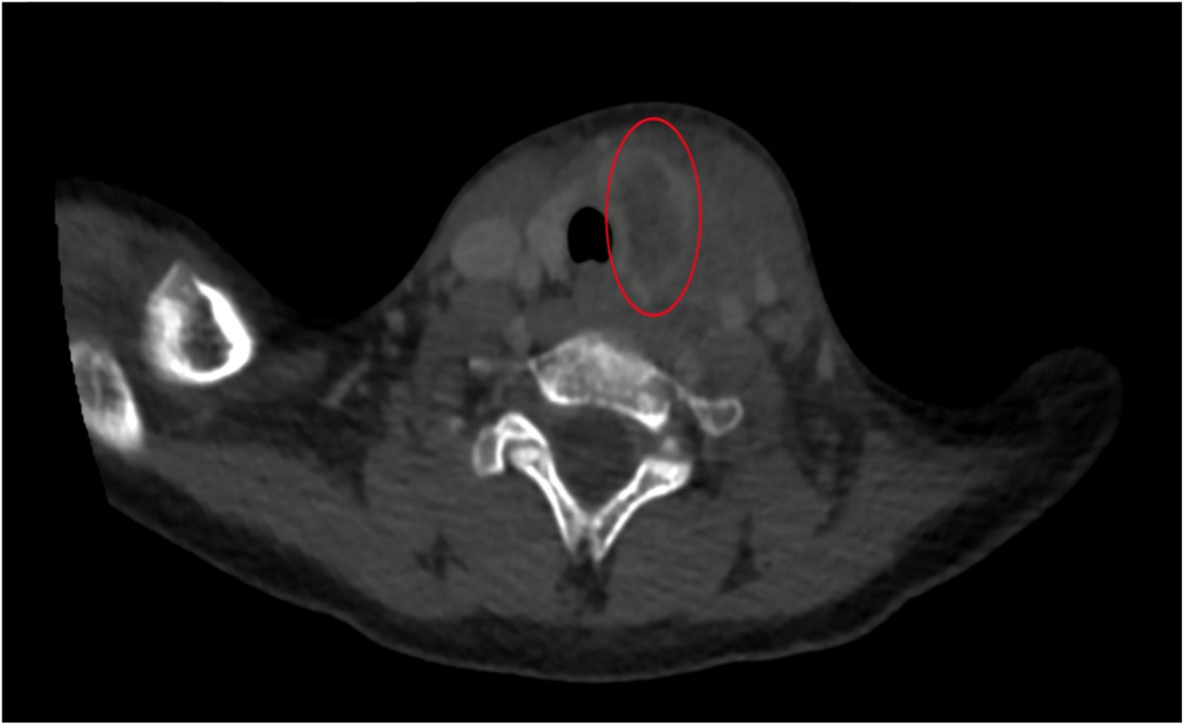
CT scan of neck depicting left lobe of thyroid replaced by hypodense lesions of fluid attenuation (red demarcated circle area); features suggestive of acute suppurative thyroiditis
